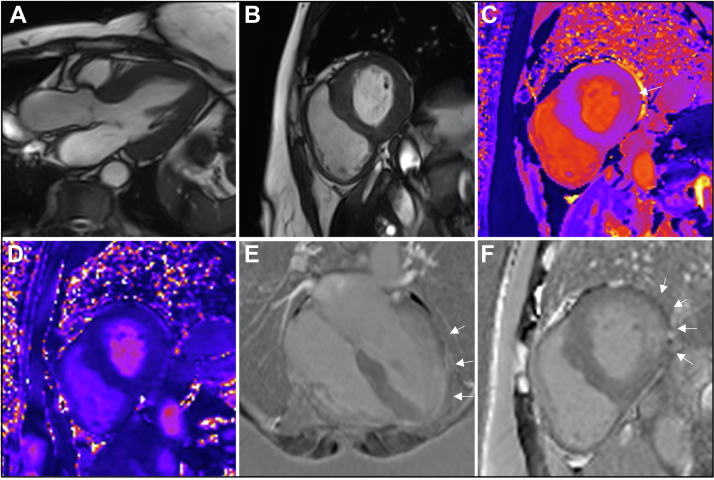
Case #1 Cardiac Magnetic Resonance Images (A) Three-chamber and (B) basal short-axis balanced steady-state free precession images. (C) Basal short-axis T_1_ map (modified look-locker inversion recovery). The arrow indicates an area of high native myocardial T_1_ relaxation time. (D) Basal short-axis T_2_ map. (E) Four-chamber phase-sensitive inversion recovery late gadolinium enhancement image acquired in the late phase following gadolinium administration. (F) Basal short-axis phase-sensitive inversion recovery late gadolinium enhancement image. The arrows indicate areas of late gadolinium enhancement. The basal short-axis T_1_ map, the basal short-axis T_2_ map, and the basal short-axis phase-sensitive inversion recovery late gadolinium enhancement images were acquired in identical imaging planes. Images are from a cardiac magnetic resonance scan conducted before the hybridized imaging.
